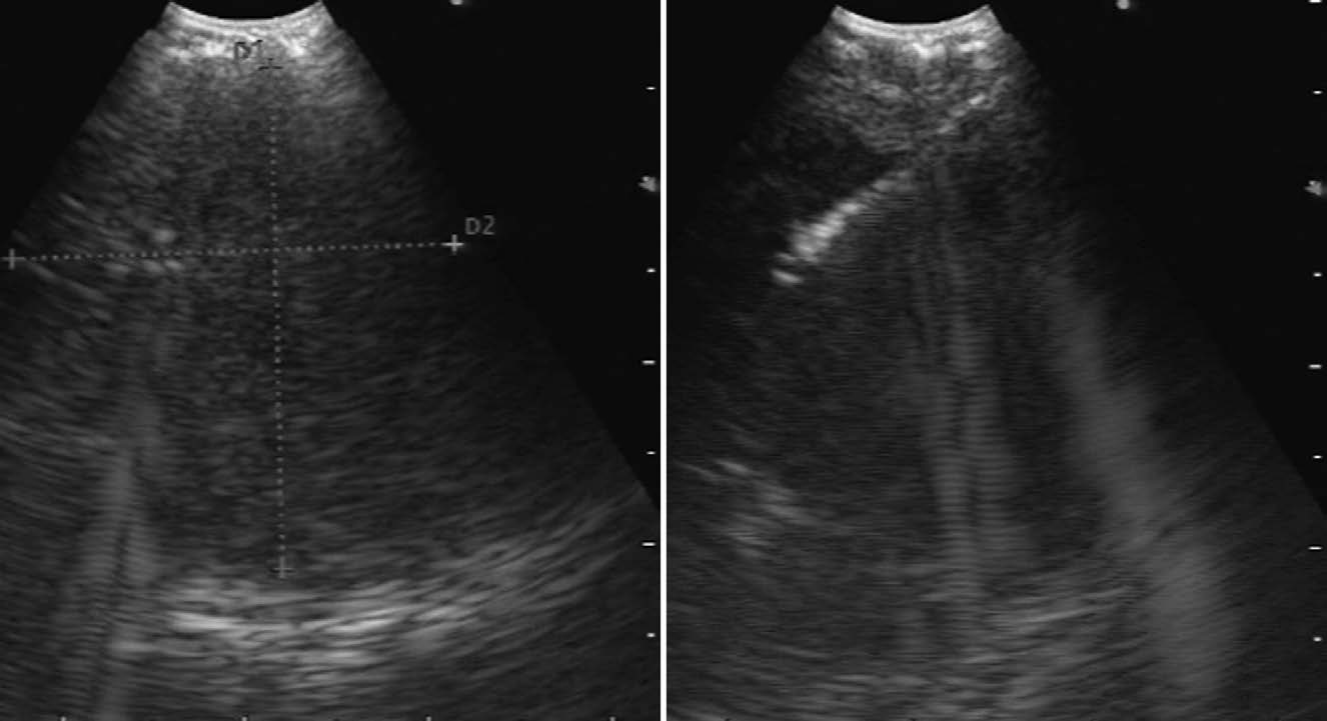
Endobronchial ultrasound revealing a well-defined, round mass with a homogeneous echogenic nature. Transbronchial needle aspiration was performed.
